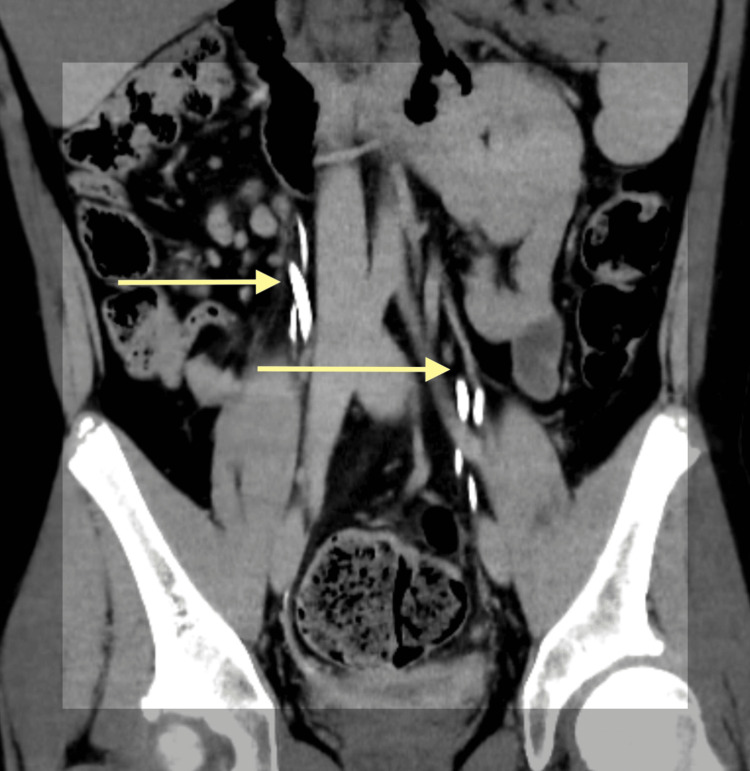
CT scan revealed a bilateral complete duplex collecting system (yellow arrow).
